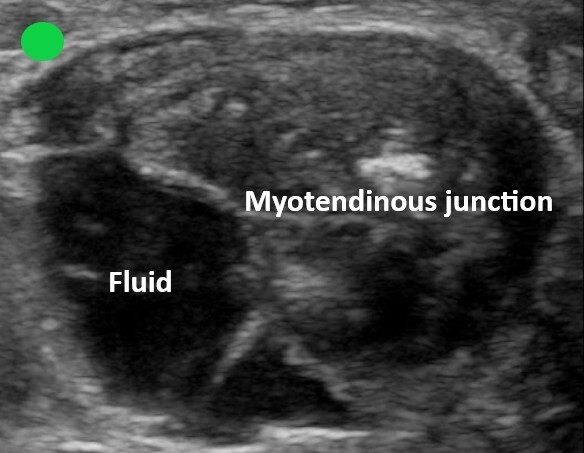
Transverse view of the Achilles myotendinous junction. Point-of-care ultrasound image obtained with a high-frequency linear probe with the probe marker directed to the left of a prone patient. A large anechoic fluid collection is present among the fibers of the myotendinous junction.
